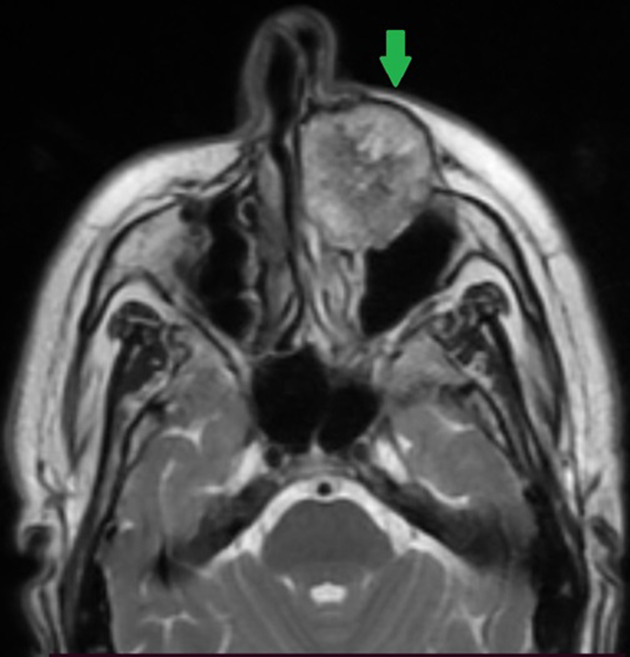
axial T2-weighted image showing a hyperintense lesion of the left maxilla (green arrow)
